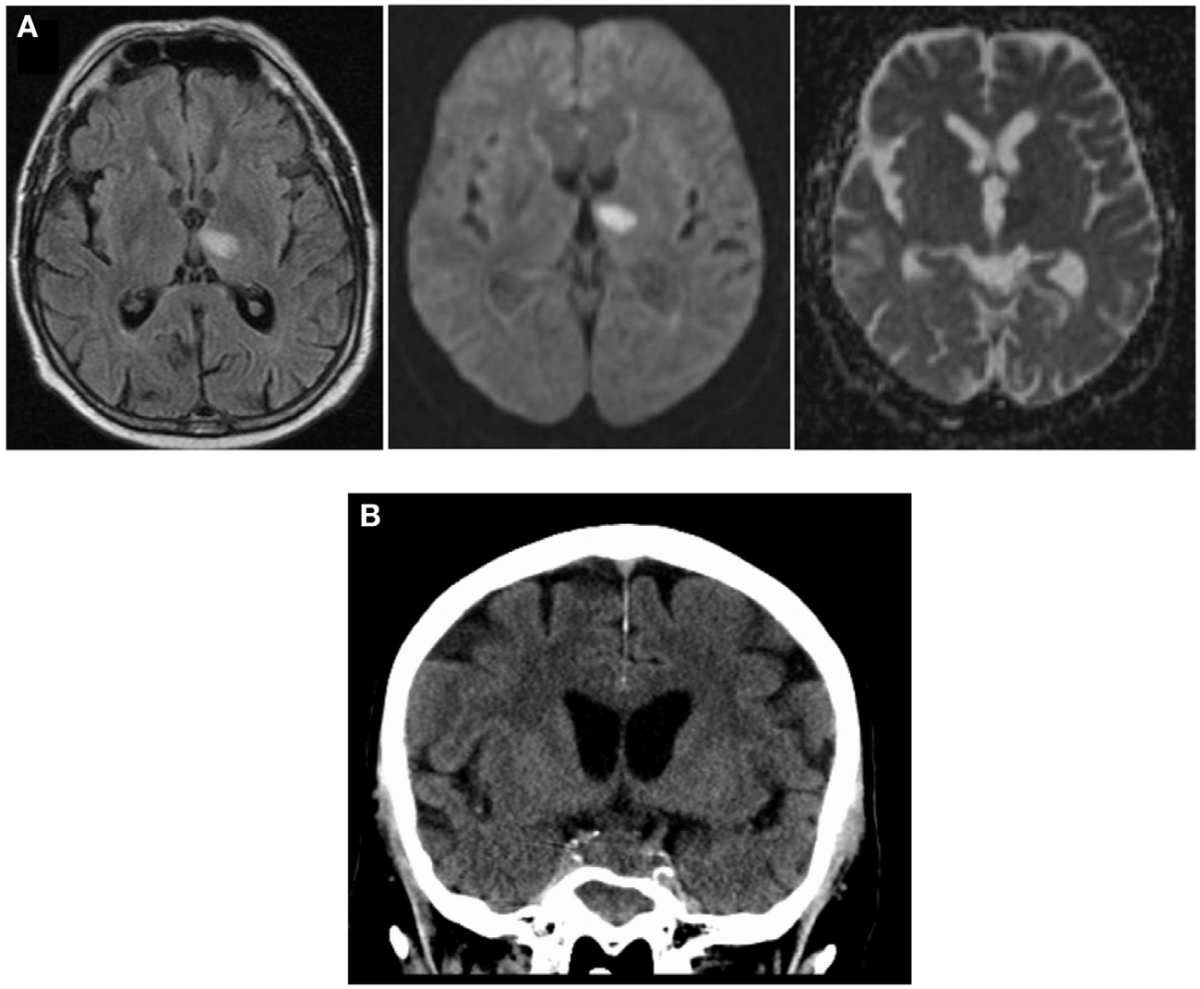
Imaging during course of disease: (A) NMR imaging on day 3 after admission shows hyperintensity in FLAIR sequence (left), high degree of diffusion-positivity (DWI, middle), and lack of shine-through effect in ADC (right), indicating acute thalamic infarction. (B) cCT scan (day 10) showing increased sellar process.
